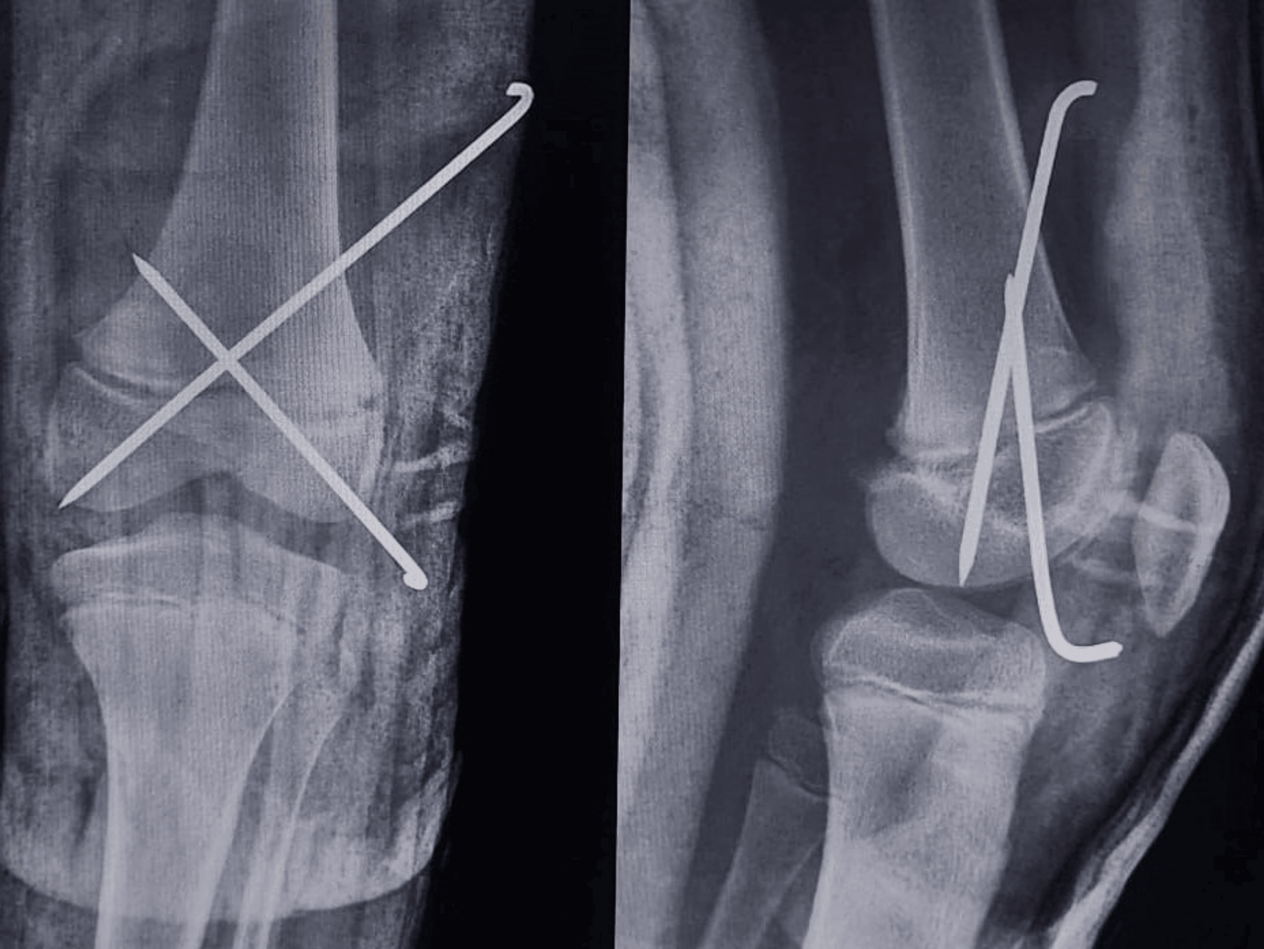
Post-operative X-ray showing anteroposterior and lateral views of the knee joint.
